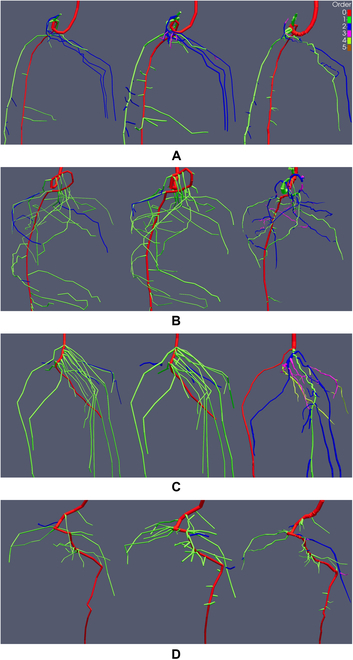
Manual tracings M (left), manual tracings after segmentation M+ (middle), and automated tracings A (right) of 4 Lupinus albus root systems (A to D) grown in sand derived by MRI scans. Reconstructions are cropped to show areas of interest. Colors display root orders, and root segments are scaled by their respective radius. Age of the root systems is between 8 and 14 d (see Table 1).
